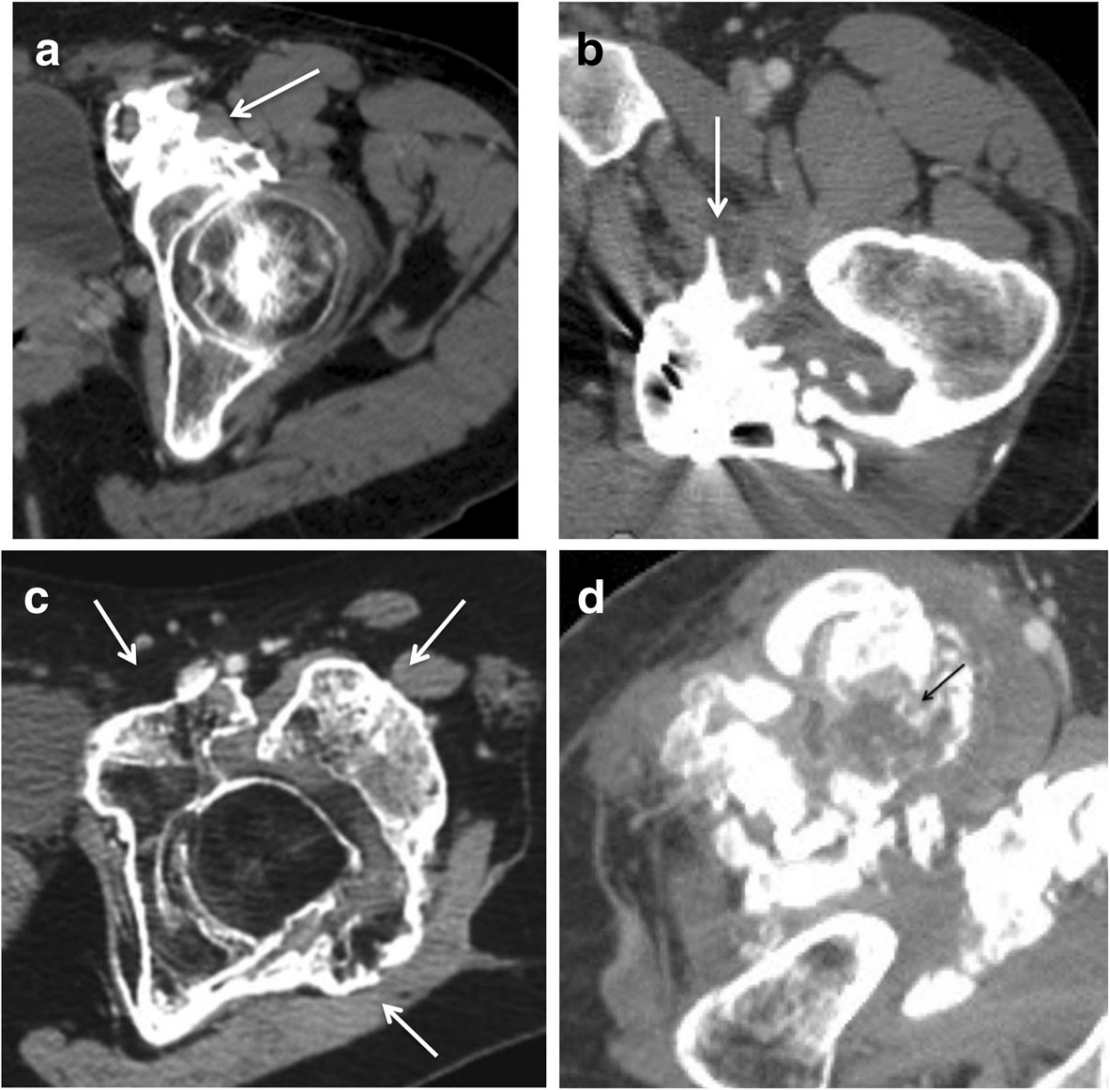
Axial CT images in four different patients. a anterior osteoma (*arrow*). b posterior osteoma (*arrow*). c circumferential osteoma. d anterior osteoma in a 31 year-old male patient. Central hypodensity with thin peripheral enhancement corresponding to an immature portion (*black arrow*)
